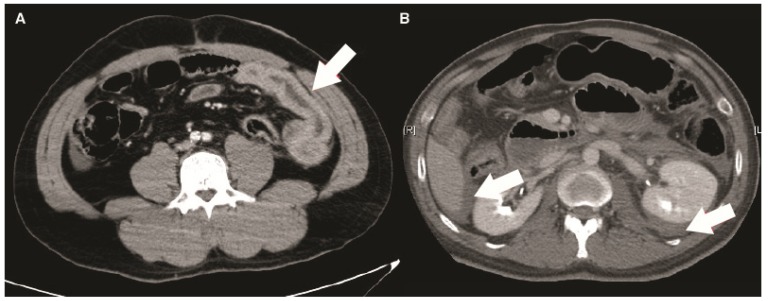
(A) The axial computed tomography revealed an abdominal bowel wall with thickened, hyperemic and fluid-filled bowel loops (arrow). (B) The image showed multiple intraperitoneal and retroperitoneal free fluid (arrows) without solid organ injury, which imply the possibility of blunt bowel and mesenteric injury.
